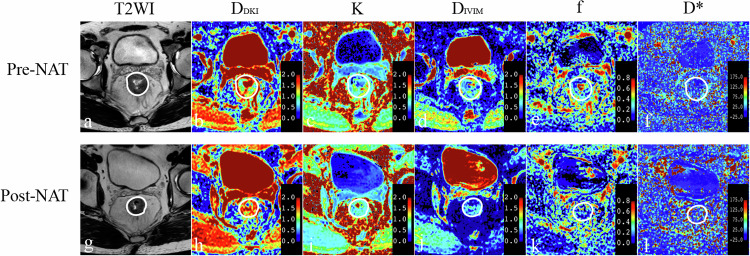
A 34-year-old man without pCR to NAT. Images in sequence are pre- and post-NAT MR imaging and IVIM–DKI parametric maps. Pre-NAT T2WI (a) and post-NAT T2WI (g) show an intermediate signal intensity rectal cancer at its largest dimension. The corresponding pre-NAT *D*_DKI_ map (b), pre-NAT *K* map (c), pre-NAT *D*_IVIM_ map (d), pre-NAT *f* map (e), pre-NAT *D*^*^ map (f), post-NAT *D*_DKI_ map (h), post-NAT *K* map (i), post-NAT *D*_IVIM_ map (j), post-NAT *f* map (k), and post-NAT *D*^*^ map (l) are shown. T2WI, T2-weighted imaging; *D*_DKI_, diffusivity of DKI; *K*, kurtosis of DKI; *D*_IVIM_, true diffusion coefficient; *f*, perfusion fraction; *D*^*^, pseudo-diffusion coefficient
